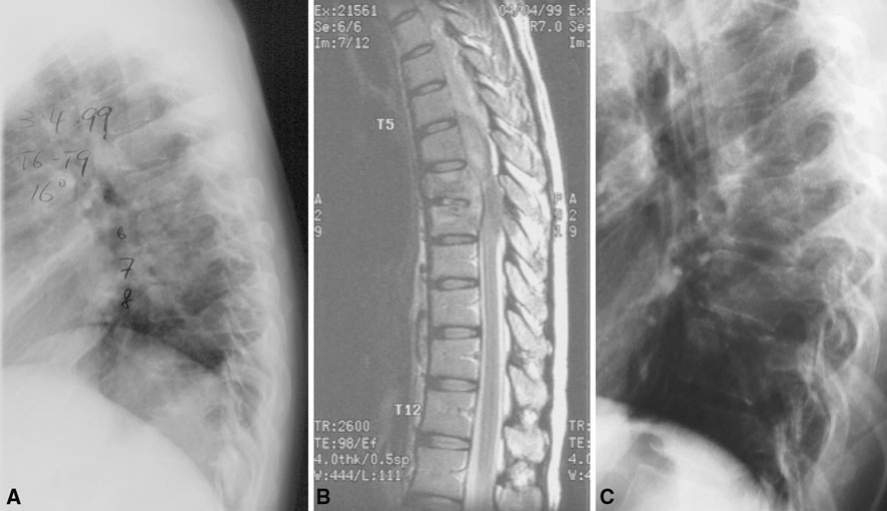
Patient was successfully treated with anti-TB medications. a, b X-ray and MRI at presentation. c X-ray 1 year after treatment showed spontaneous fusion
